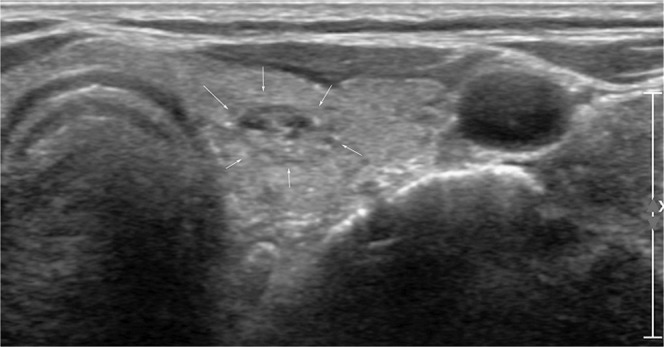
An ultrasonography (US) image of a left thyroid nodule in a 77-year-old woman who was confirmed with cancer in the right thyroid gland. A 1-cm sized isoechoic nodule with internal echogenic spots was seen (arrows). Four radiologists (1 faculty, 1 fellow, and two residents) interpreted the nodule as cancer. In contrast, 3 CNN-combinations interpreted it as benign. The nodule was diagnosed as adenomatous hyperplasia.
